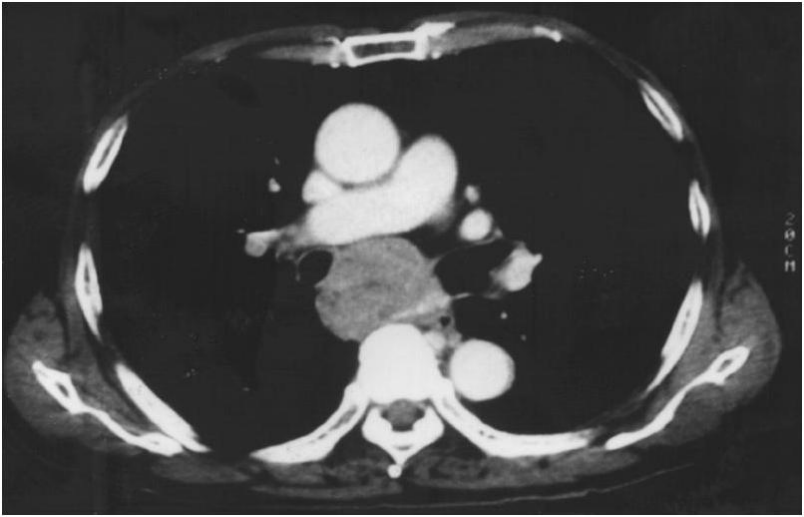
Chest CT image before radiation therapy. A bulky mediastinal lymph node metastasis was detected.
